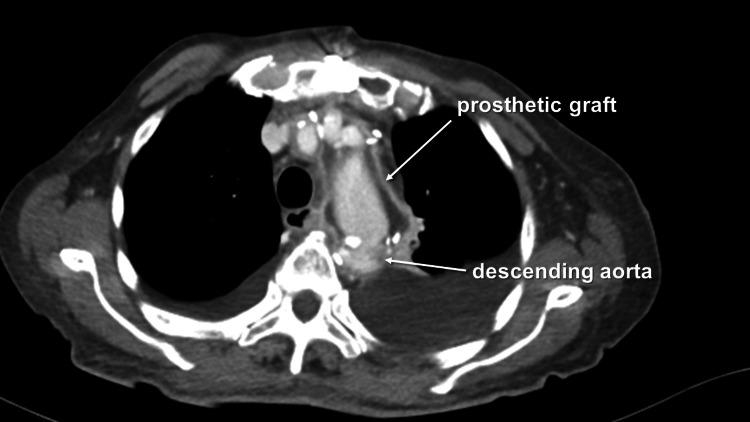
Postoperative enhanced computed tomography revealing no signs of infection recurrence
